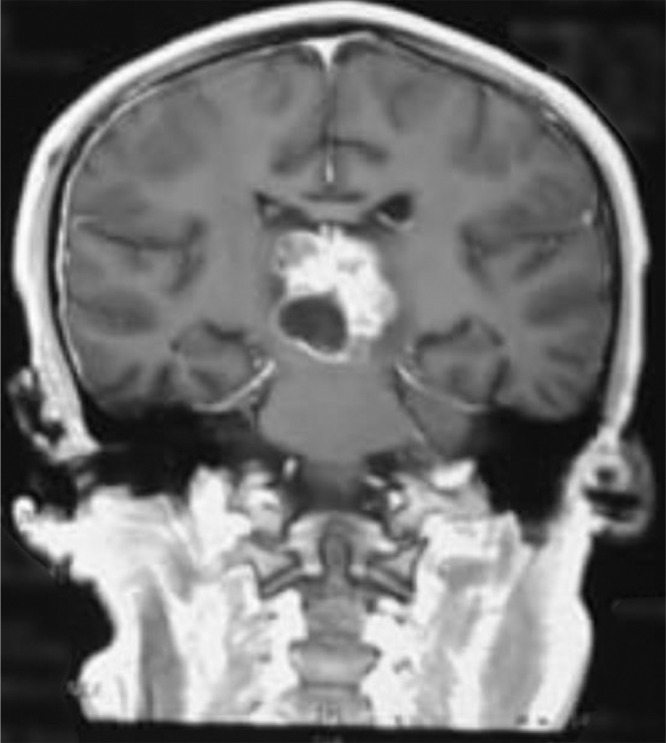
MRI T1 of the head with contrast (coronal section).
